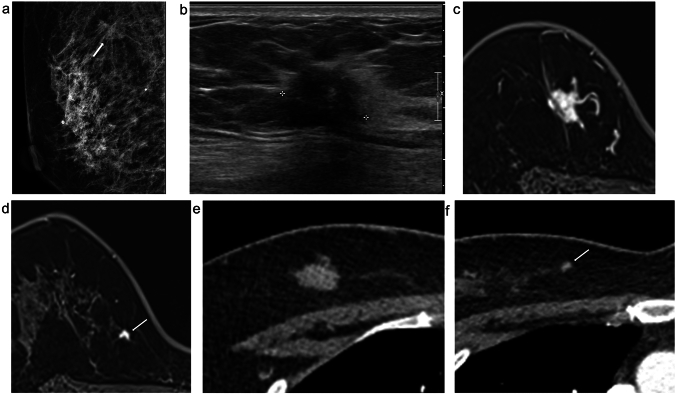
A 48-year-old woman with invasive ductal carcinoma, clinical stage 2 A (ARL No.4); an additional suspicious lesion not seen on US was confirmed on CT, and ARL was applied. a Mediolateral oblique view mammography reveals an irregular, spiculated, isodense mass (arrow) in the upper posterior aspect of the right breast. b US shows a 1.7 cm irregular hypoechoic mass in the right breast. c, d MRI displays an irregular enhancing mass confirmed to be malignant. An additional enhancing mass (arrow) measuring 0.5 cm is visible in the lower inner aspect of the confirmed malignant lesion. A second-look US was performed on this lesion, but it could not be differentiated by US. e, f Both the confirmed malignant lesion and an additional suspicious mass (arrow) are visible on CT. ARL was applied to both lesions, and surgery was conducted; both lesions were ultimately diagnosed as invasive breast carcinoma
